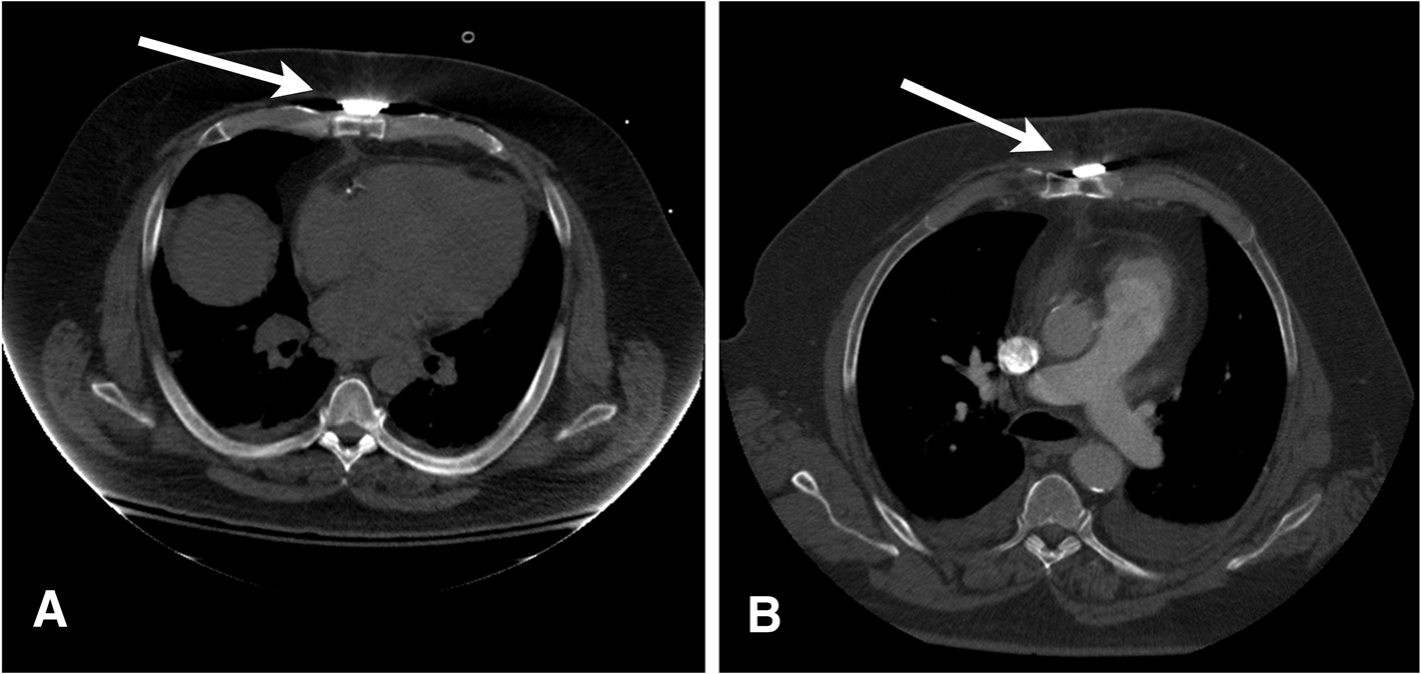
a. Chest computed tomography scans obtained from 2 different patients, taken 1 week (a) and 1 month (b) after placement of a sternal plate (arrows)
